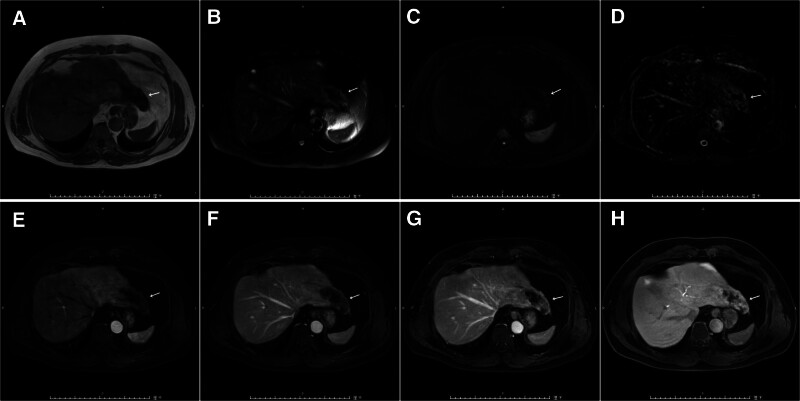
MRI manifestations of AH in the left lobe of the liver. (A) T1WI and (B) T2WI with fat suppress. (C) DWI (b = 800 s/mm²). (D) ADC map. (E) Arterial phase. (F) Portal venous phase. (G) Delayed phase. (H) Hepatobiliary phase. ADC = apparent diffusion coefficient, AH = anastomosing hemangioma, DWI = diffusion-weighted imaging, MRI = magnetic resonance imaging.
